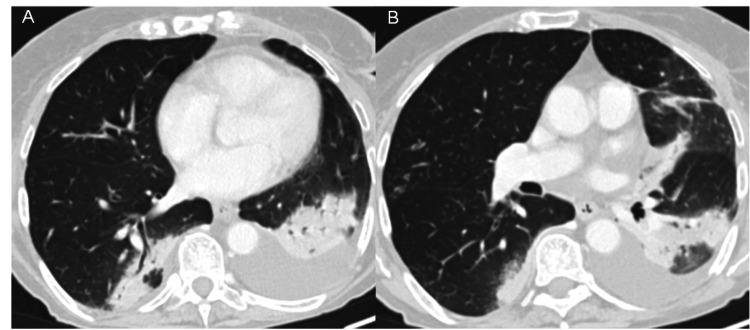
Chest CT at admission. Extensive bilateral consolidation foci, ground-glass-opacification areas in the left superior lobe, left inferior lobe and right inferior lobe, and pleural effusion.
